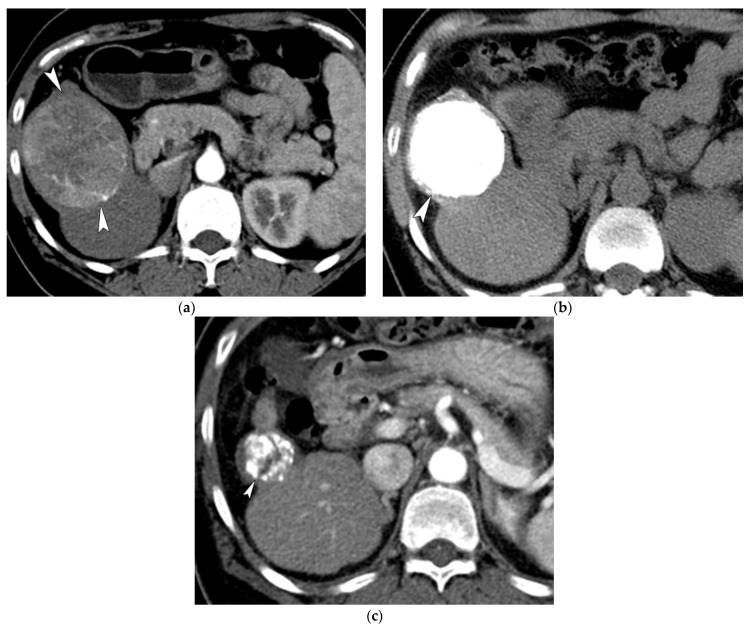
Images of a 47-year-old female patient with HCC. (a) Contrast-enhanced axial CT image in the arterial phase shows a large enhancing mass (7 cm in maximal diameter; arrowheads). (b) Non-enhanced axial CT image obtained 1 month after the initial TACE shows the presence of compact lipiodol uptake in the tumor and a small decrease in tumor size (5 cm; arrowhead). (c) CT at 5 years after initial TACE shows a further decrease in tumor size without recurrence (3 cm; arrowhead).
